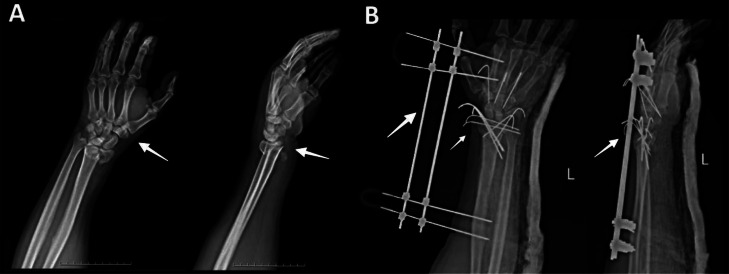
Radiographic assessment of distal radius fracture. (A) Anteroposterior (AP) and lateral X-ray views showing comminuted distal radius fracture (white arrows) with articular involvement and displacement. (B) Postoperative AP and lateral X-ray views demonstrating external fixator placement and K-wire fixation (white arrows) for management of the distal radius fracture and carpal-metacarpal injuries.
